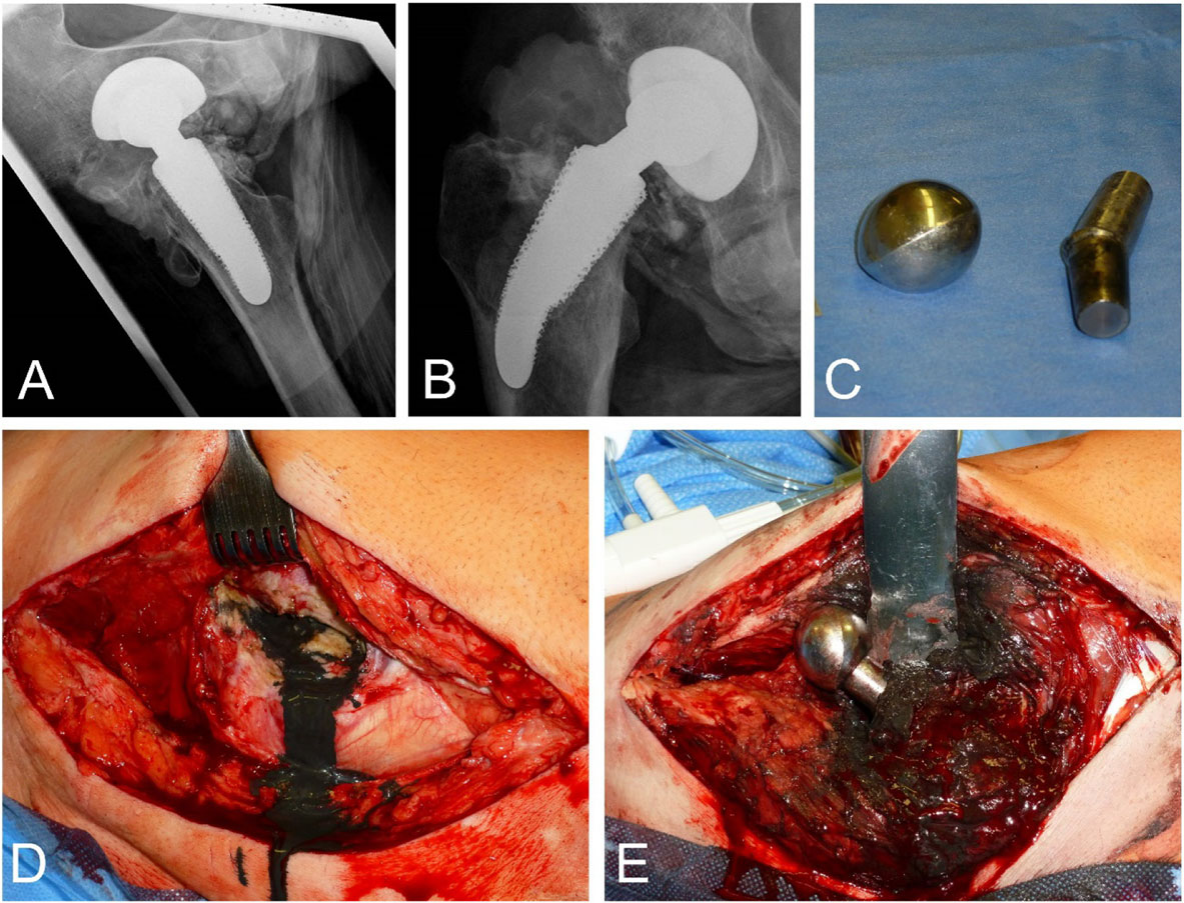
X-ray of the right hip joint in two planes: A) mediolateral projection and B) anterior-posterior projection show a decentered prosthesis head as evidence of inlay wear but no evidence of periprosthetic fracture or material loosening. Pronounced, periarticular heterotopic ossification (A) with bridging of the joint space (grade 4 according to Brooker). Flaw changes in the density of the peri-prosthetic region hint toward heavy metal abrasion. Streaky calcifications consistent with myositis ossificans (especially seen in A) in projection to the adductors and rotators indicate local inflammation probably related to metal abrasion. C Explanted femoral head and neck. Note the massive wear of the head, which was round at the time of implantation. D Leakage of black synovial fluid after removal of the hip joint capsule. E Massive metallosis and synovialitis after luxation of the hip joint. Abrasion of the femoral head of the prosthesis
